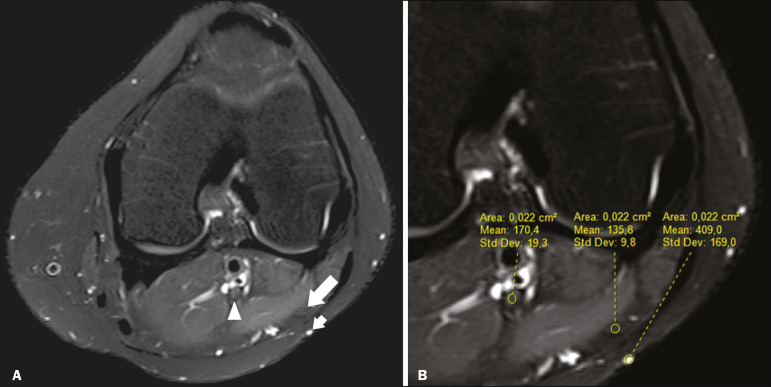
Signal intensity in the CPN and SV. A: Axial T2-weighted MRI sequence with fat saturation of a normal knee, showing the CPN (long arrow), TN (arrowhead), and SV (short arrow). B: The same image magnified for signal measurement in the area of interest. In this case, the CPN/TN and CPN/SV signal ratios were 0.79 and 0.33, respectively.
